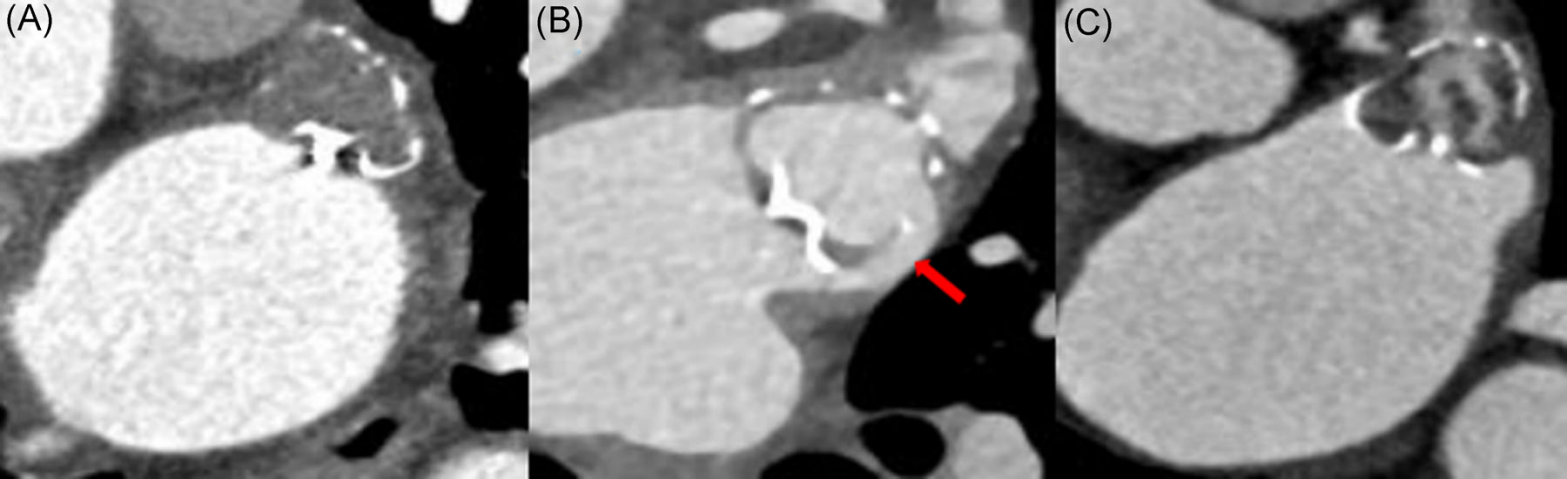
CT images showing complete LAA closure by Watchman device (A), peri‐device leak (B), and trans fabric leak (C). Panel (A) shows no evidence of contrast enhancement within the Watchman device or beyond its edges. Panel (B) shows peri‐device leak (red arrow) with contrast traversing around the device into the distal portions of the LAA. Panel (C) shows an incomplete device seal with evidence of contrast uptake within the device but not at its proximal border, suggesting residual permeability of the fabric. CT, computed tomography; LAA, left atrial appendage
